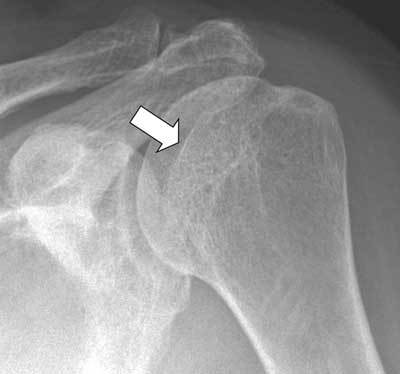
Frontal radiograph of shoulder showing double contour of humeral head (“trough sign”) (arrow) due to an reverse Hill-Sachs lesion secondary to history of posterior dislocation.
